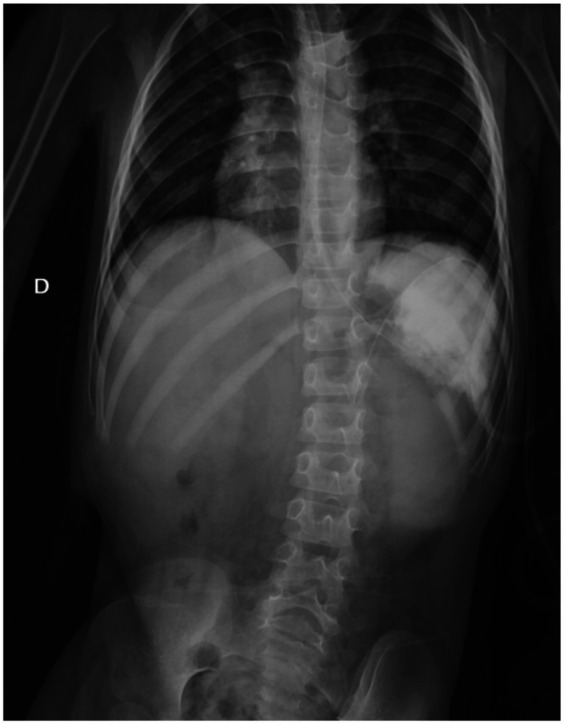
Plan abdominal x-ray after aspiration and UGI contrast showed partial rotation of the greater curvature toward the diaphragmatic side.
